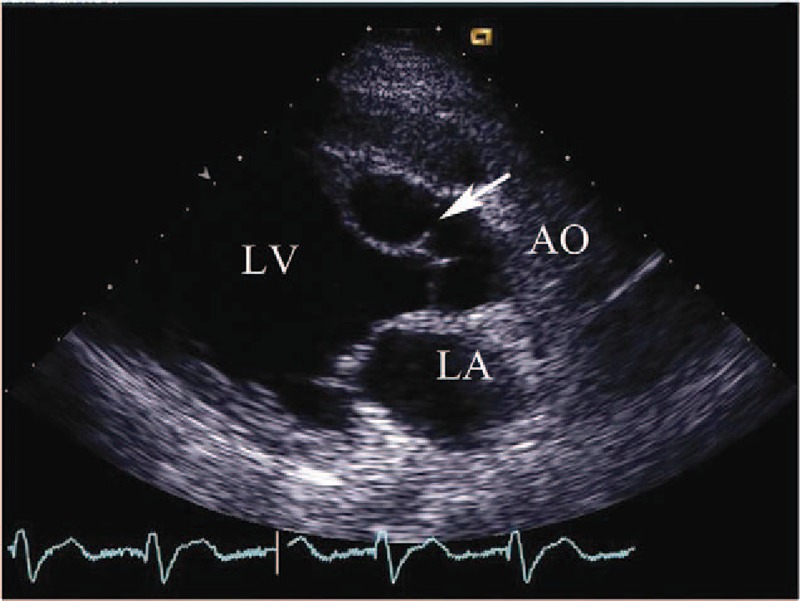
(Patient #2) Transthoracic echocardiogram (parasternal long axis view) showed the right sinus of Valsalva aneurysm and the entry site of rupture (arrow) into the dissecting cystic-like cavity in the interventricular septum. AO = aorta, LA = left atrium, LV = left ventricle.
